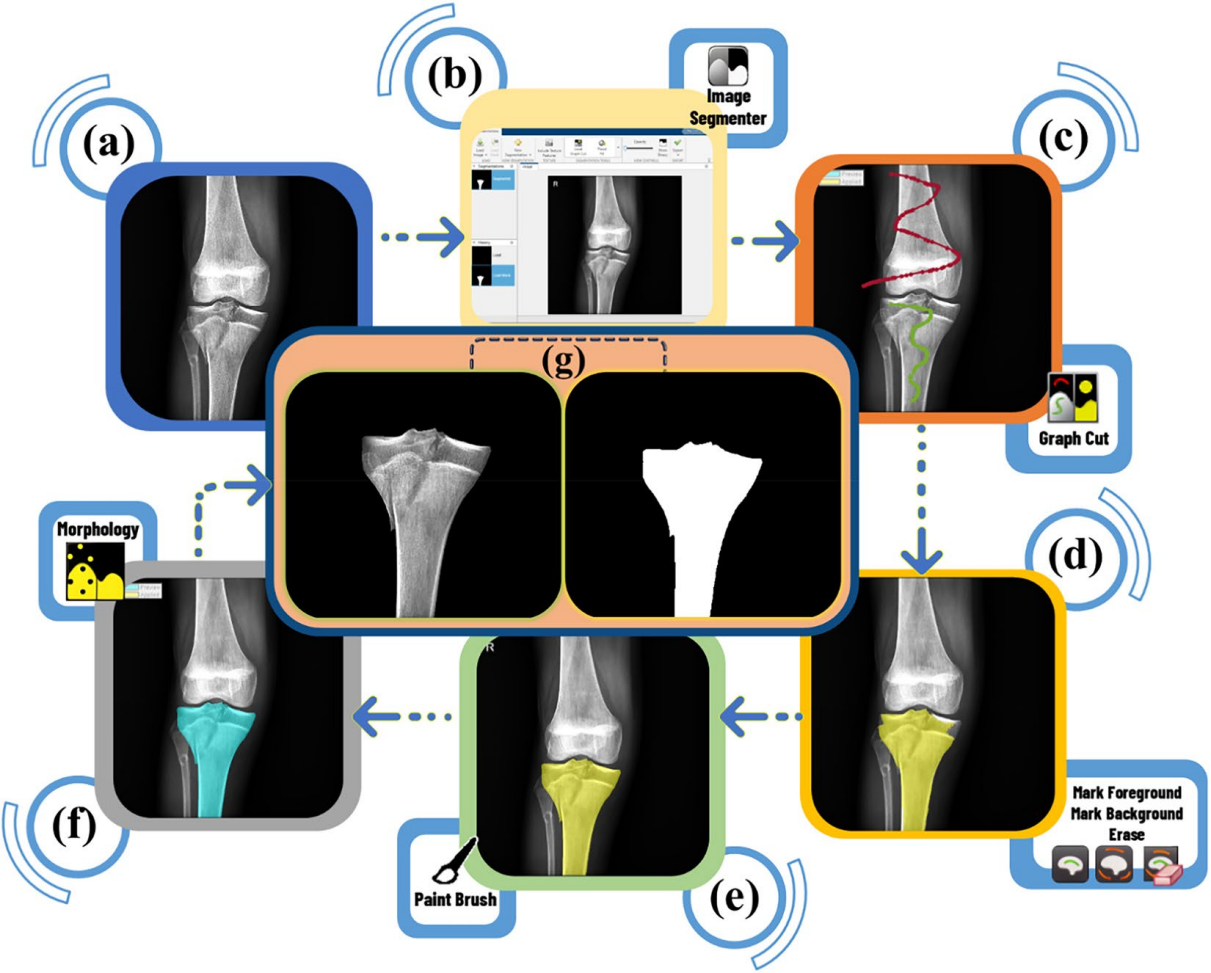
The steps of tibia segmentation in the MATLAB Image Segmenter app. (a) AP knee radiography, (b) Importing image to Image Segmenter app, (c) Applying Graph Cut, (d) Primary mask from the segmented portion after specifying foreground and background of the image (the foreground is the area we wish to segment, while the background is everything else), (e) New mask after applying the paint-brush tool to modify the edges of the mask and fulfill Tibia, (f) Final mask, in morphology tab, we apply certain operations on the mask, such as dilation and erosion, by opening and closing on the mask pixels, (g) Export masked image and binary mask of X-ray image to the MATLAB workspace.
